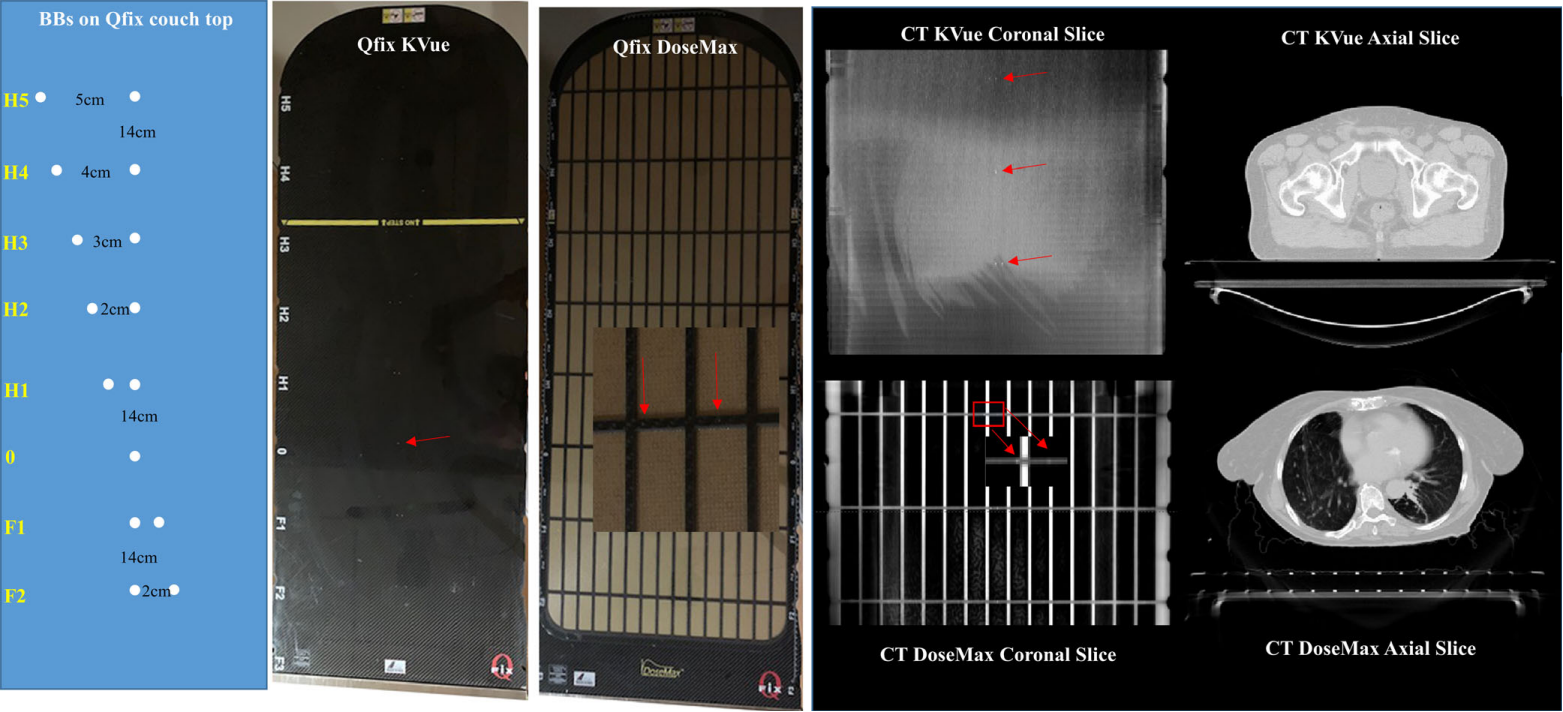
Qfix kVue and DoseMax couch‐top. From left to right: scheme of BBs on a couch‐top, Qfix kVue couch‐top, DoseMax couch‐top, computed tomography coronal and axial images for both couch‐tops.
